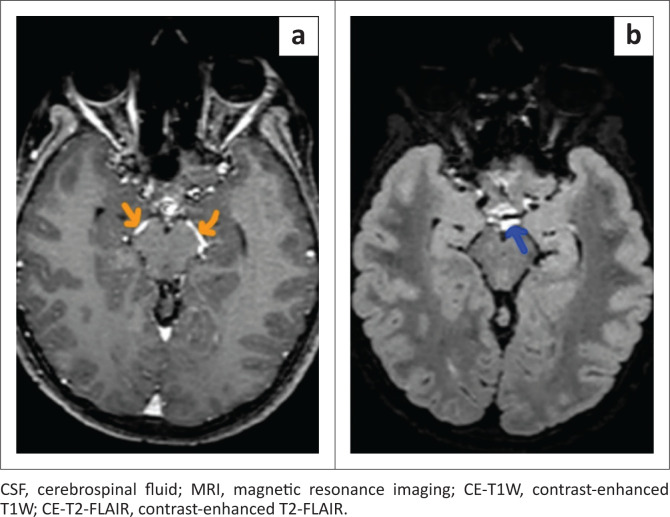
Axial brain MRI of a 15-year-old female negative for meningitis on CSF analysis. (a) CE-T1W shows enhancing vessels (orange arrows) around the midbrain. (b) CE-T2-FLAIR shows false positive basal cisternal enhancement (blue arrow) anterior to the midbrain.
